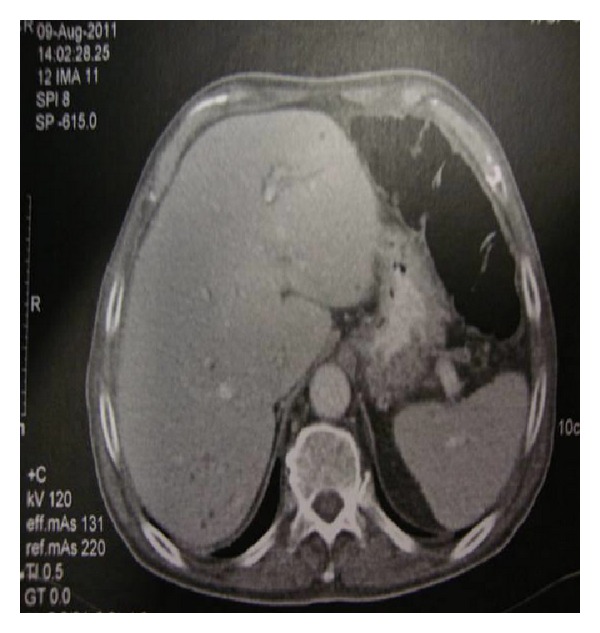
Abdominal CT showing hypodense lesions located in the liver and spleen and enlarged retroperitoneal lymph nodes.
